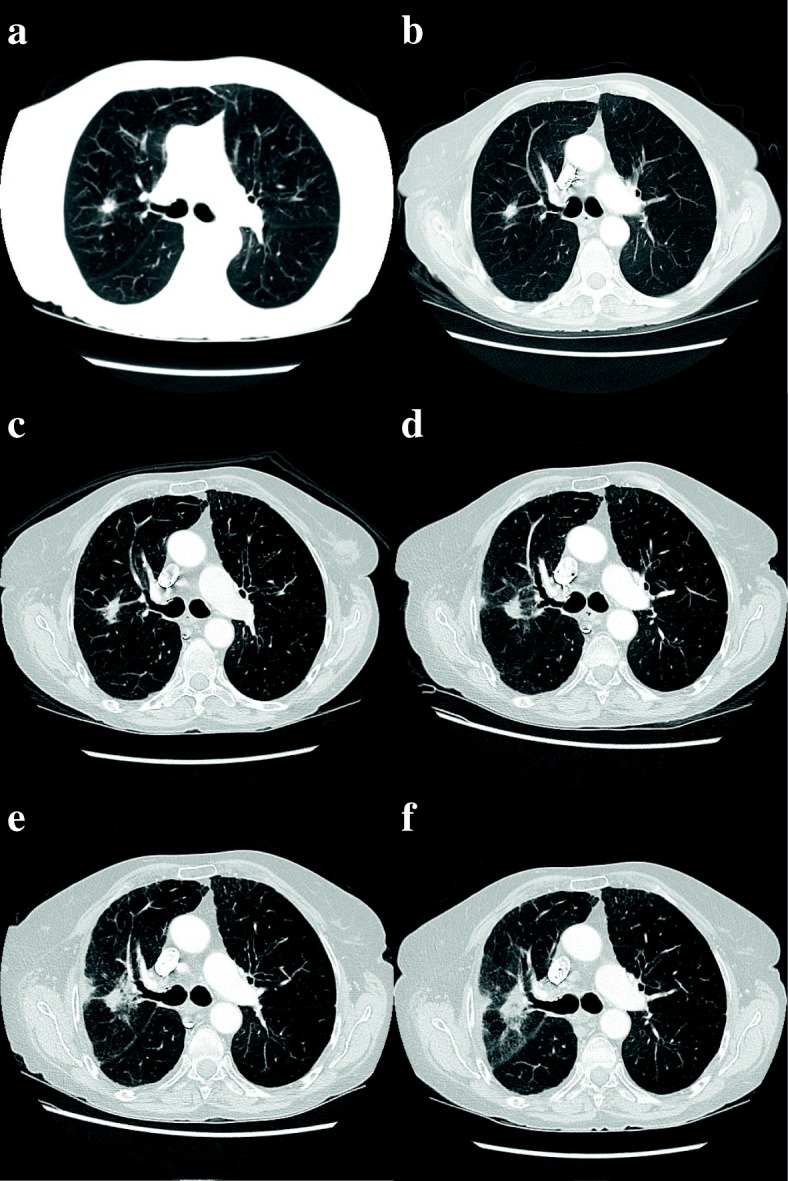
CT images demonstrating the primary lesion a pre-treatment, b 3 months, c 6 months, d 9 months and, e 12 months and f 15 months post-treatment. Evolving post-radiation changes are observed
